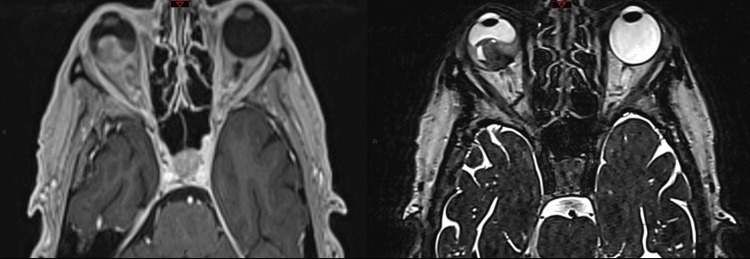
Magnetic Resonance Image - T1 (left), T2 (right). The pre-treatment magnetic resonance images demonstrate the endophytic intraocular mass without evidence of extraocular expansion.
